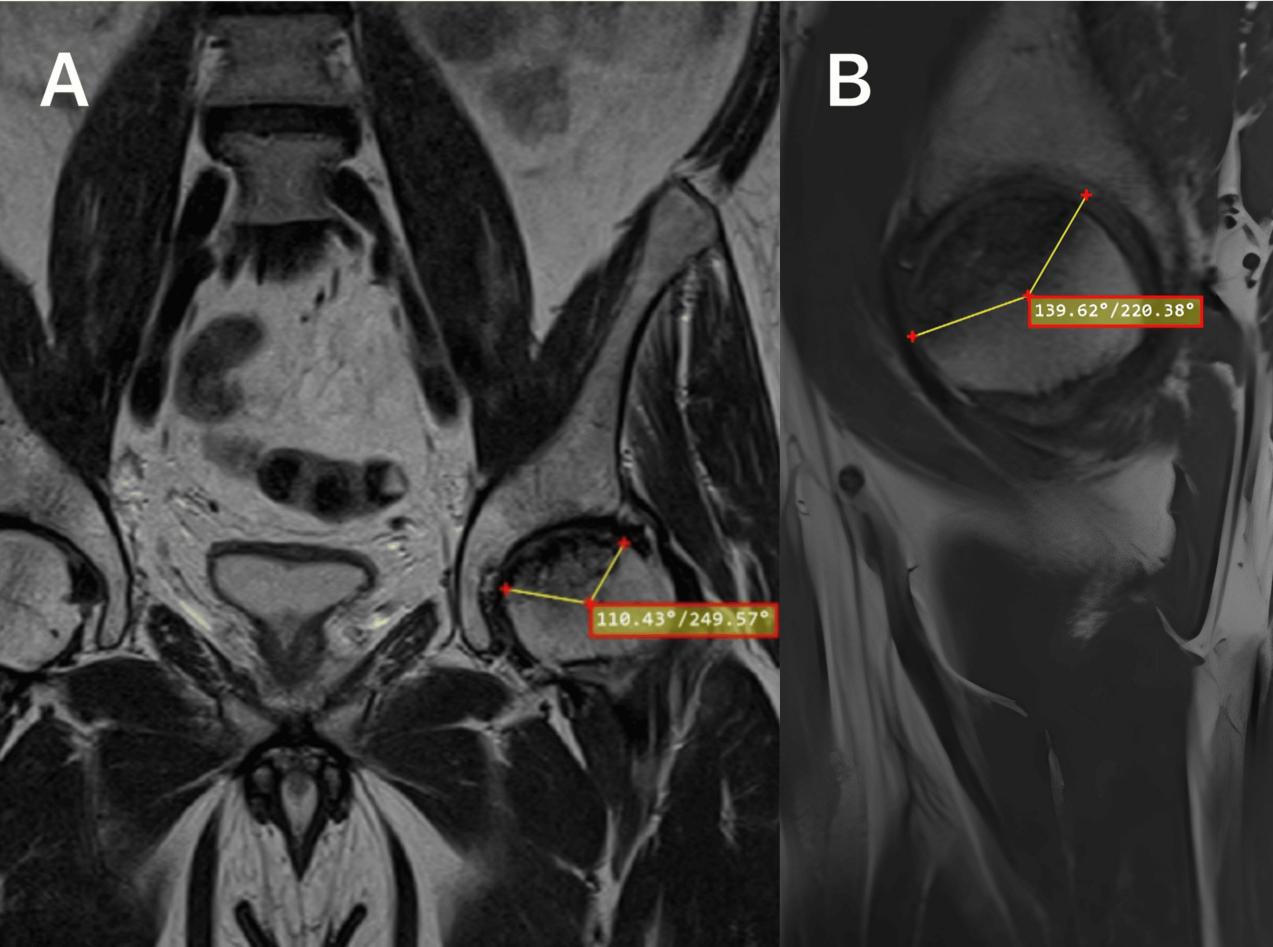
An example of the Cherian method, which estimates lesion size using angles (yellow lines) measured on MRI images showing maximum lesion size in (A) coronal and (B) sagittal slices
